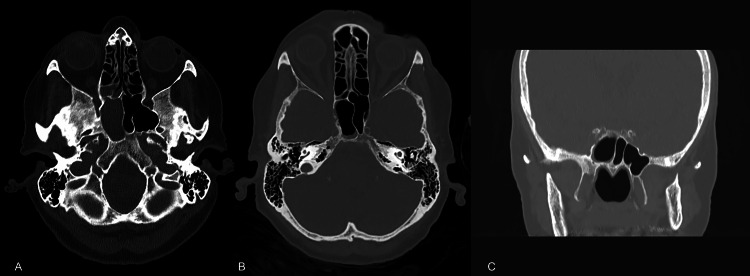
CT scan findings A: axial and coronal CT scans showing the greatest extent of right sphenoid sinusitis early after initial presentation; B: after antibiotic treatment two months later; B, C: bony erosion in the lateral sinus wall despite the finding of treated sinusitis.
